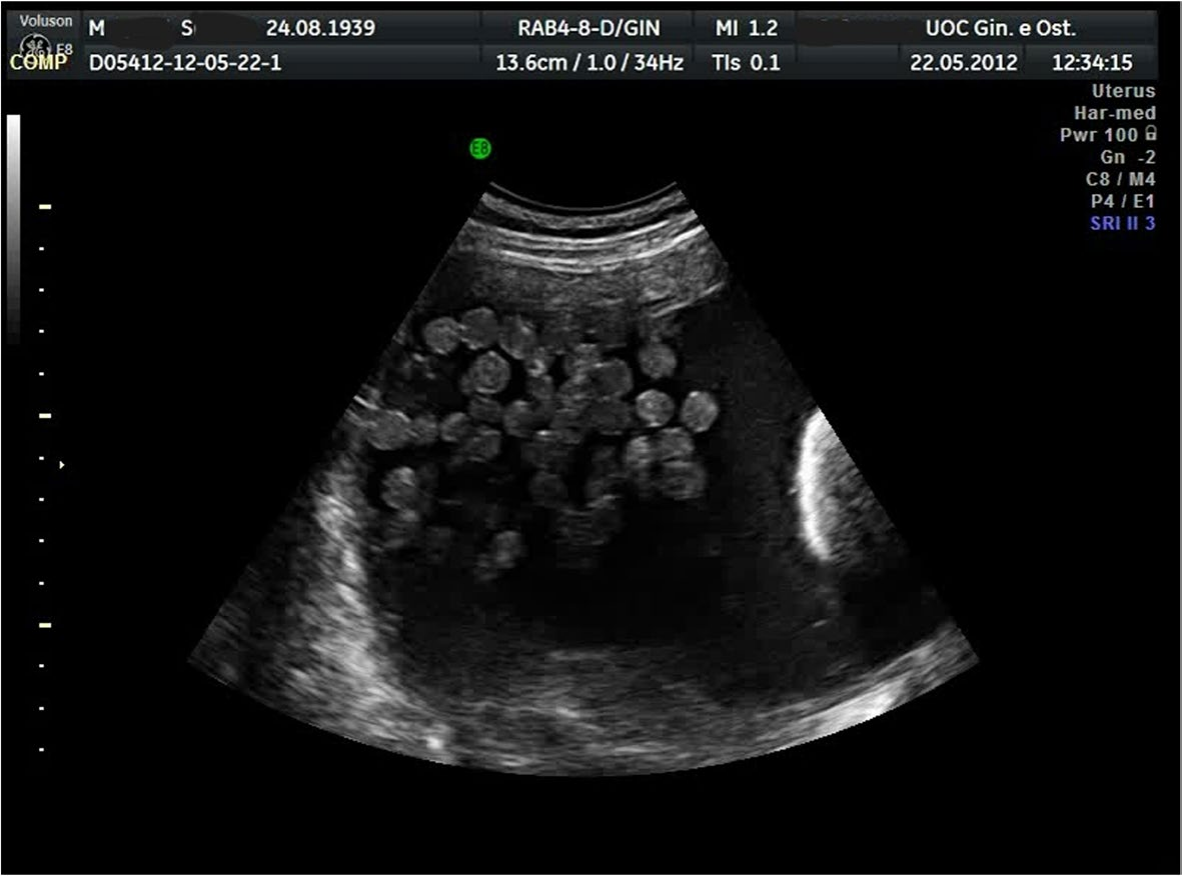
Initial ultrasound examination with evidence of pelvic mass consisting of uniloculated cyst of about 24 cm of maximum size with multiple small mobile hyperechoic internal lesions. An uneven area of about 2 cm located in the inferior cystic wall is also highlighted
